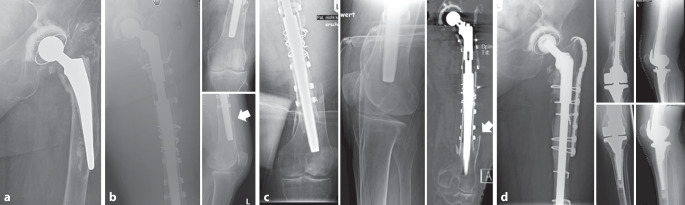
84 Jahre, weiblich. a Aseptisch gelockerte zementierte Prothese (Primärimplantation vor 17 Jahren) mit distaler Schaftspitzenmigration und Destruktion der lateralen Femurkortikalis und konsekutiver Fraktur (Vancouver-Typ B3). Rarefizierte Knochenstruktur. b Postoperative Kontrolle nach zementfreiem, modularem Schaftwechsel mit additiven Cerclagen. Bereits hier ist distal die periprothetische Anschlussfraktur (*Pfeil*) ersichtlich. c Postoperative Röntgenkontrolle 16 Tage nach der initialen Revisionsoperation. Zunehmende Dislokation (*Pfeil*) der Fraktur (Röntgen und CT). d Postoperative Kontrolle nach erneuter Revision mit Wechsel auf eine zementierte Durchsteckprothese mit Implantation einer gekoppelten Knieendoprothese sowie additiver Krallenplattenosteosynthese. Postoperativ schmerzadaptierte Vollbelastung der Extremität
